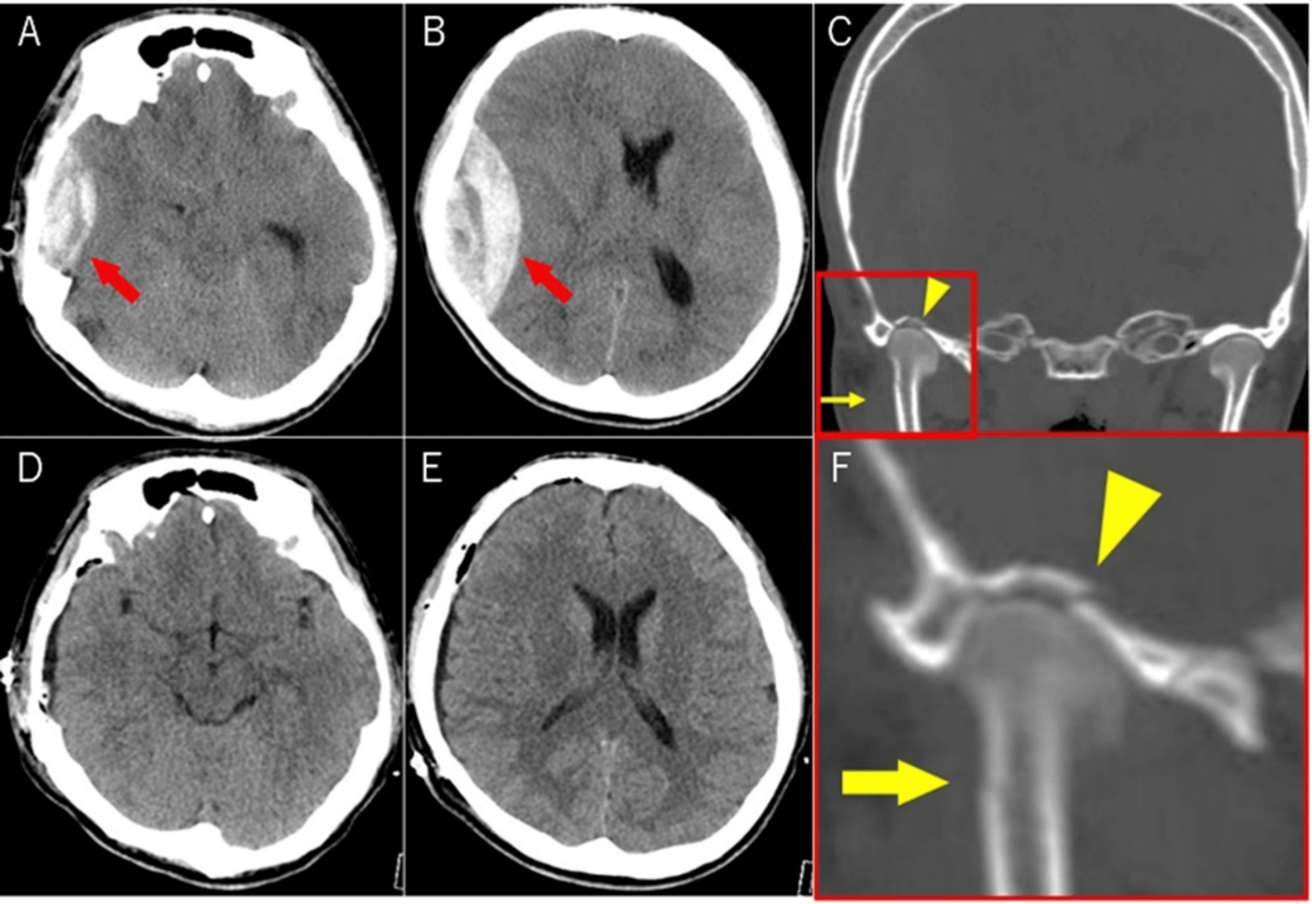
Computed tomography of the head at the time of presentation and postoperatively (A, B) The red arrow indicates acute epidural hematoma from the right middle cranial fossa to the temporal convexity area. (C) shows fracture lines in the right mandibular process (yellow arrow) and mandibular fossa (yellow arrowhead). (D, E) postoperative images. (F) is the magnified image of the fracture site. No fracture line is seen in the convexity area. The operation removed almost all hematoma.
